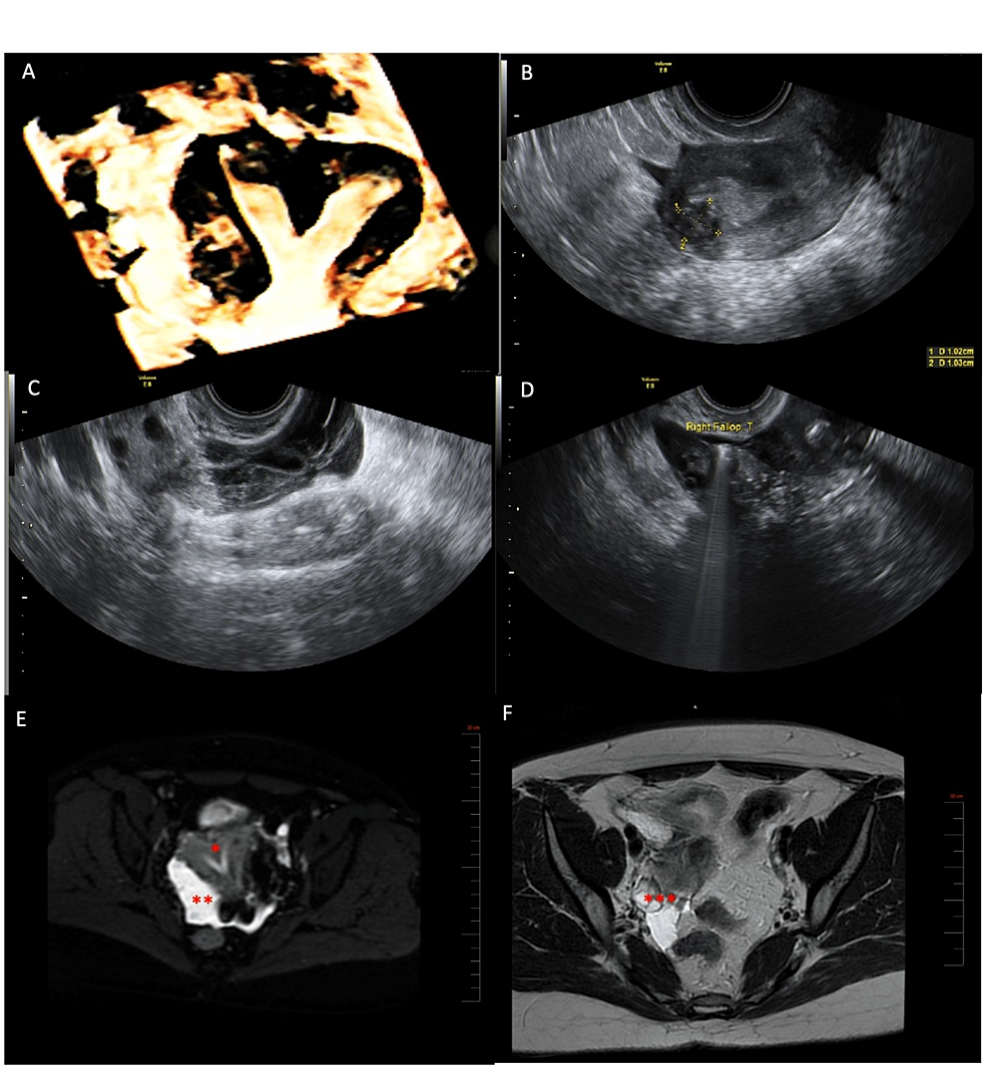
(A) 3D ultrasound of the uterus showing the uterine septum and the type I fibroid. (B) 2D ultrasound of the uterus showing the uterine fibroid. (C) Heterogeneous fluid next to the right ovary mimicking a hematosalpinx. (D) Patent right fallopian tube on sonohysterosalpingography. (E) MRI showing septated uterus (*) and suspicion of right-sided hydrosalpinx (). (F) MRI showing the right ovary (*) surrounded by fluid accumulation. MRI: magnetic resonance imaging
